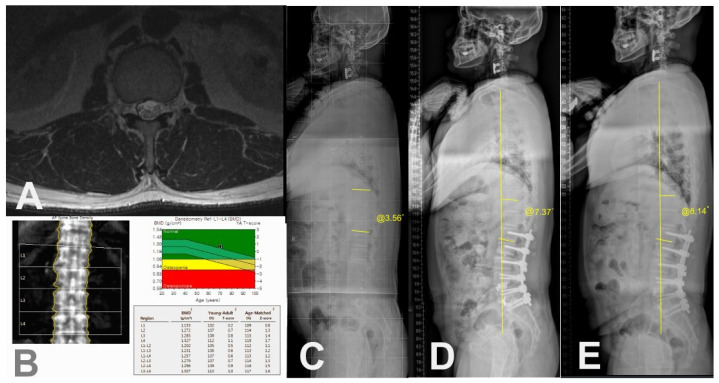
A case of a 73-year-old female patient without PJK. (A) Preoperative axial T2-weighted magnetic resonance image showing erector spinae muscle with minimal fat infiltration at T12-L1 level. (B) Preoperative BMD showing no osteoporosis. (C) Preoperative whole spine standing lateral radiograph showing PJA of 3.56°. (D) Postoperative 3-month whole spine standing lateral radiograph showing PJA of 7.37°. (E) Postoperative 3-year standing lateral whole spine radiograph showing PJA of 8.14° with maintained C7SVA.
